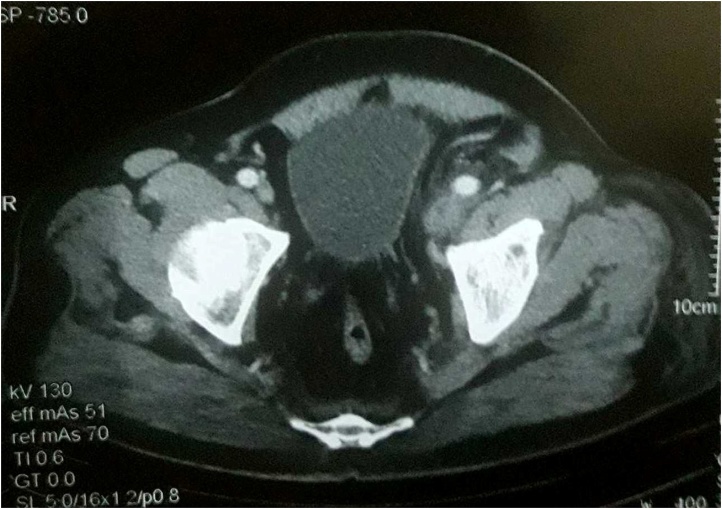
Axial CT scan showing left femoral vein thrombosis.
